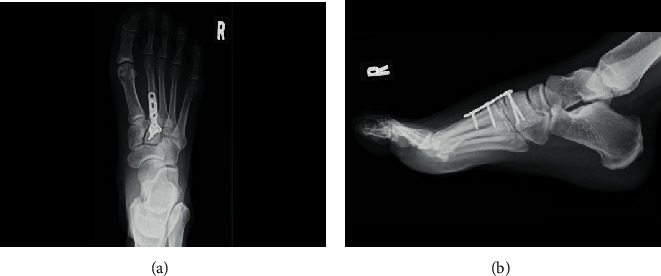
Postoperative (a) anteroposterior and (b) lateral foot radiographs. The nonunion site was fixed using the bridging plate fixation technique with a locking plate, which was placed between the distal fragment of the second metatarsal and the medial cuneiform.
